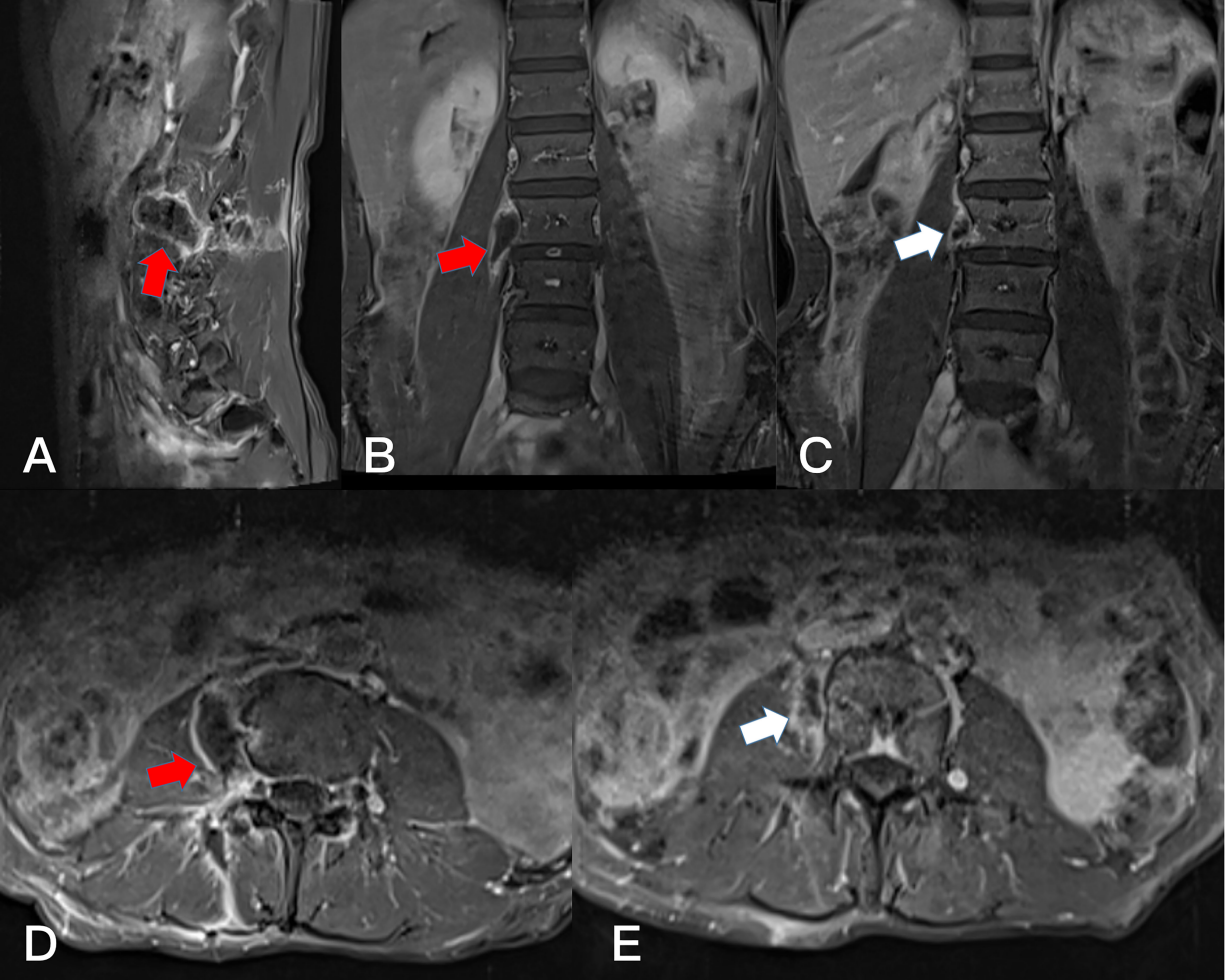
Postoperative T1-weighted enhanced MRI on sagittal (A), coronal (B), and axial (D) plane. Resection of the dumbbell tumor could not be identified after 1 month (arrows). Postoperative T1-weighted enhanced MRI on coronal (C), and axial (E) plane. Resection of the dumbbell tumor could not be identified after 1 year (arrows).
